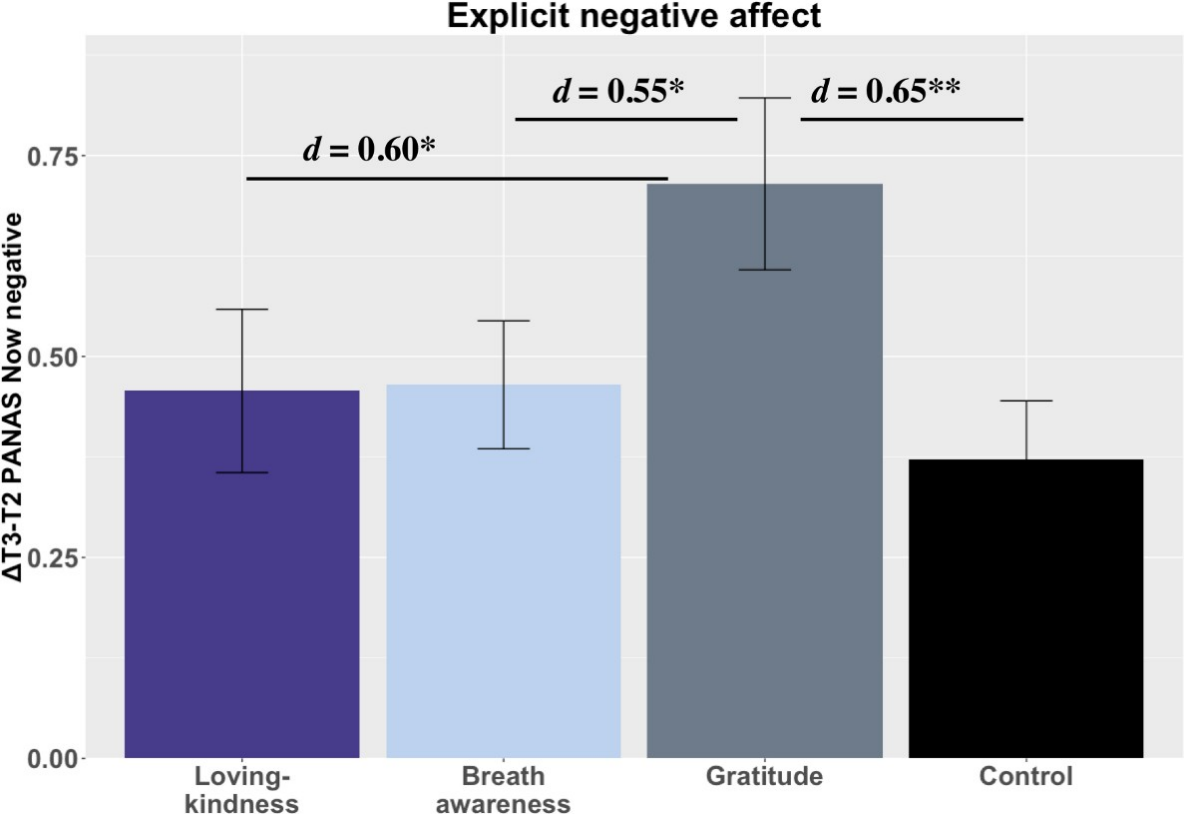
Cold pressor effects on negative affect. Notes: * = *p* < .001, * = p* < .01,  = p <* .05, *t = p* < .10; 4A. Loving-kindness = LK; Breath awareness = BA; Gratitude = GT; Control = CT.
